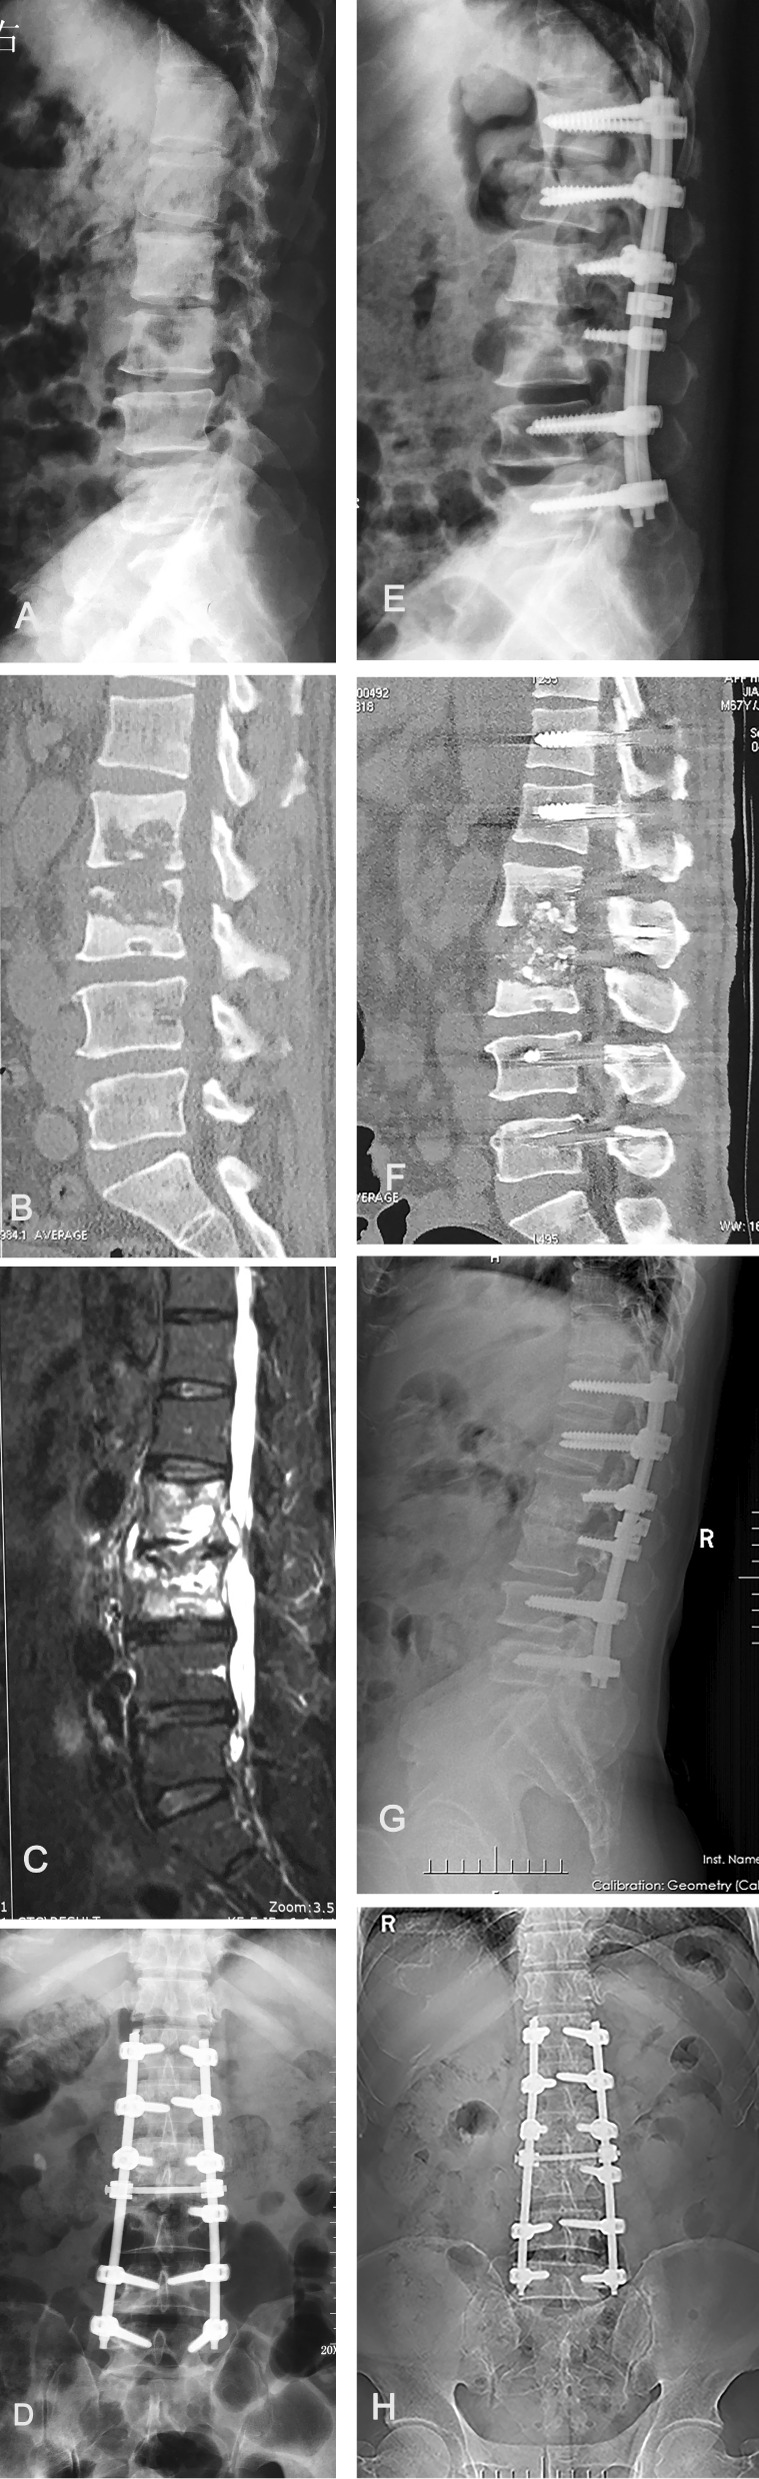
A 39-year-old female with L4–5 tuberculosis. a The lateral X-ray film before surgery demonstrated intervertebral stenosis between the L4–5. b, c Preoperative CT and MRI showed that L4–5 vertebral bone destruction with paravertebral abscess. d–f Postoperative X-ray and CT of a patient who patient underwent posterior internal fixation from L2 to S1 and structural iliac bone graft at L4–5. g, h X-ray at 24 months postoperative showing bone fusion between L4 and L5, without signs of tuberculosis recurrence
